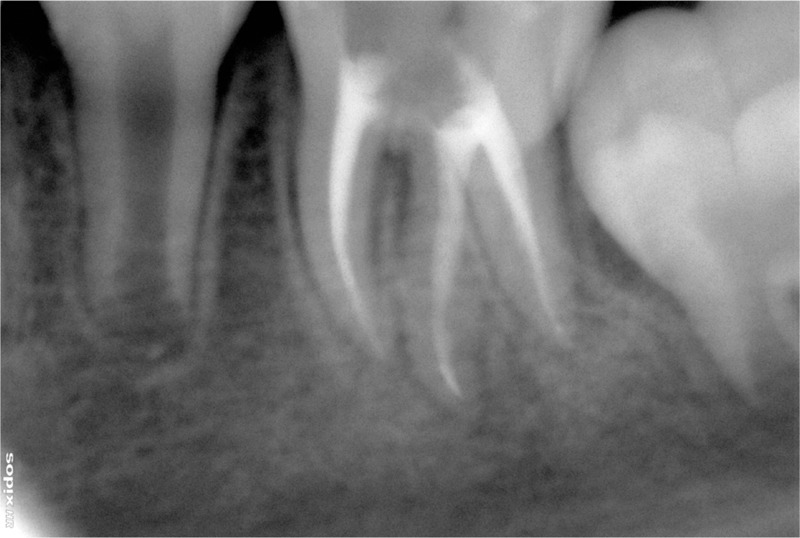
Postoperative radiograph revealed tooth 36 after root canal therapy (RCT). The periapical radiolucent area around the distal root apex has reduced. RCT = root canal therapy.
